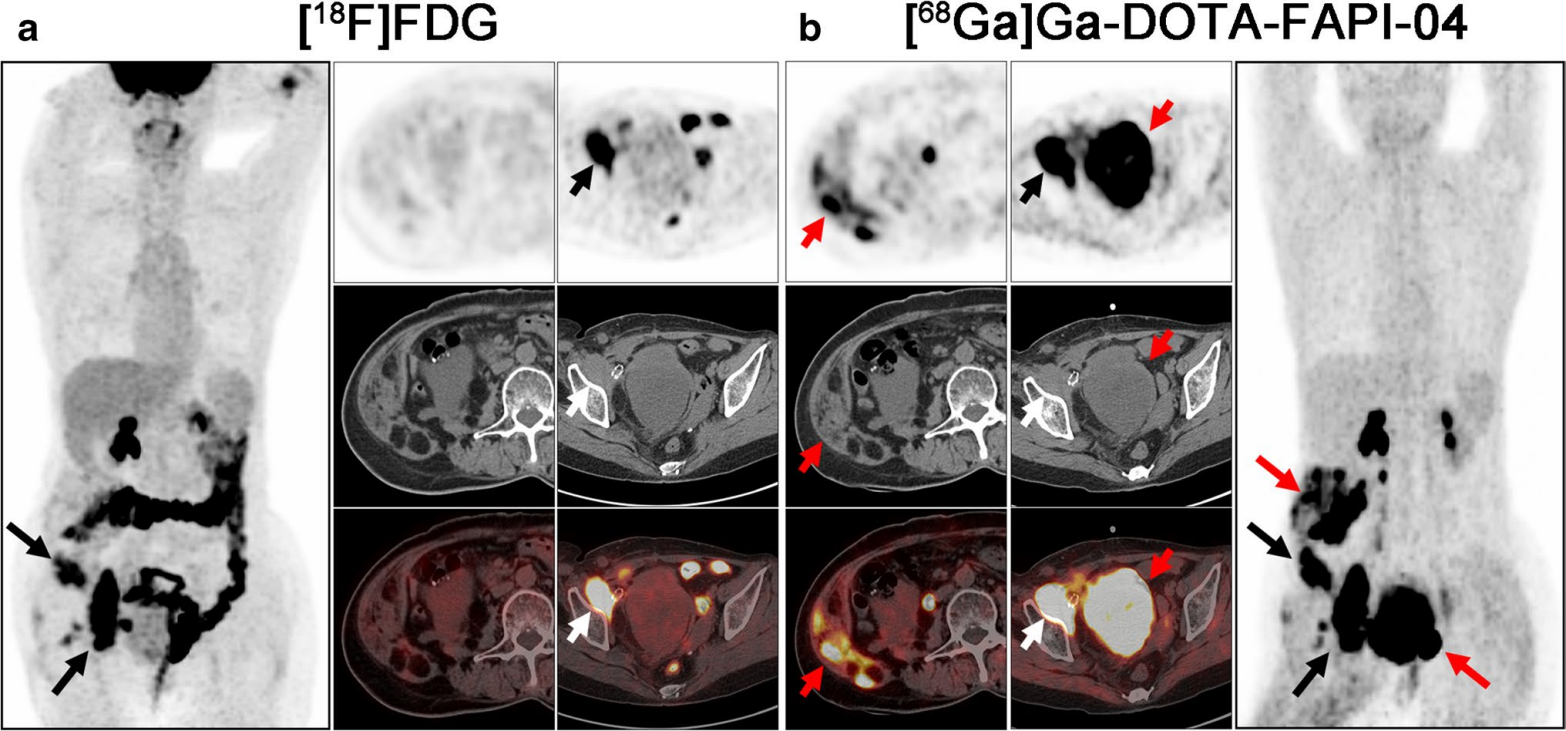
A 64-year-old woman (patient #8) pathologically confirmed with retroperitoneal well-differentiated liposarcoma received radical operation 4 years ago. [^18^F]FDG PET/CT (a) demonstrated pelvic wall metastatic foci with intensive metabolic activity. But the abdominal wall foci and large pelvic metastatic foci showed no intensive uptake of [^18^F]FDG. Compared with [^18^F]FDG, [^68^ Ga]Ga-DOTA-FAPI-04 PET/CT (b) detected all the metastatic lesions with intense [^68^ Ga]Ga-DOTA-FAPI-04 activity. Black and white arrows indicated the tumor lesions detected by both tracers, and red arrows indicated the tumor lesions detected by [^68^ Ga]Ga-DOTA-FAPI-04 alone
